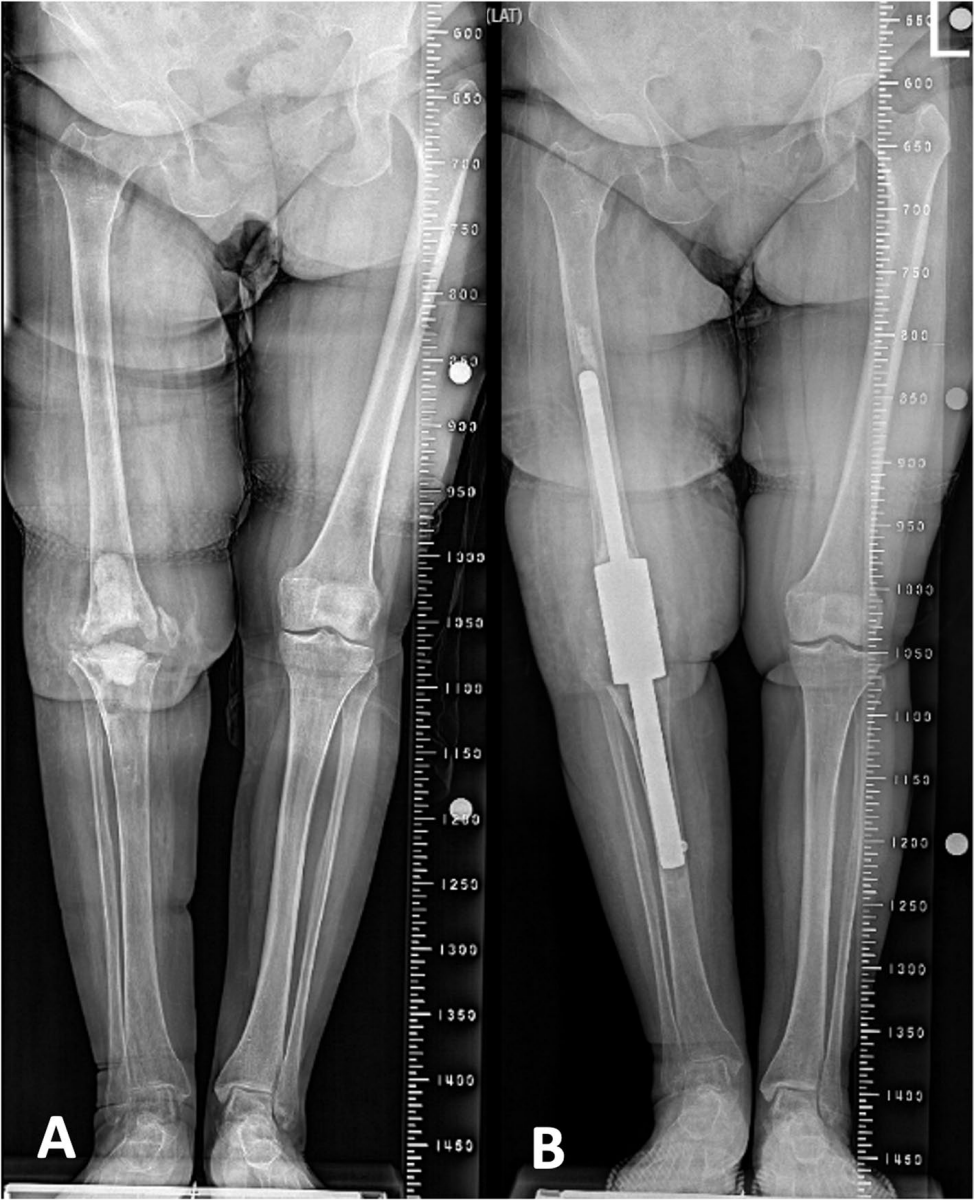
Pre-operative (a) and post-operative (b) full-length radiographs of a patient who underwent knee arthrodesis with a modular knee arthrodesis sytem after uncontrolled periprosthetic knee infection
